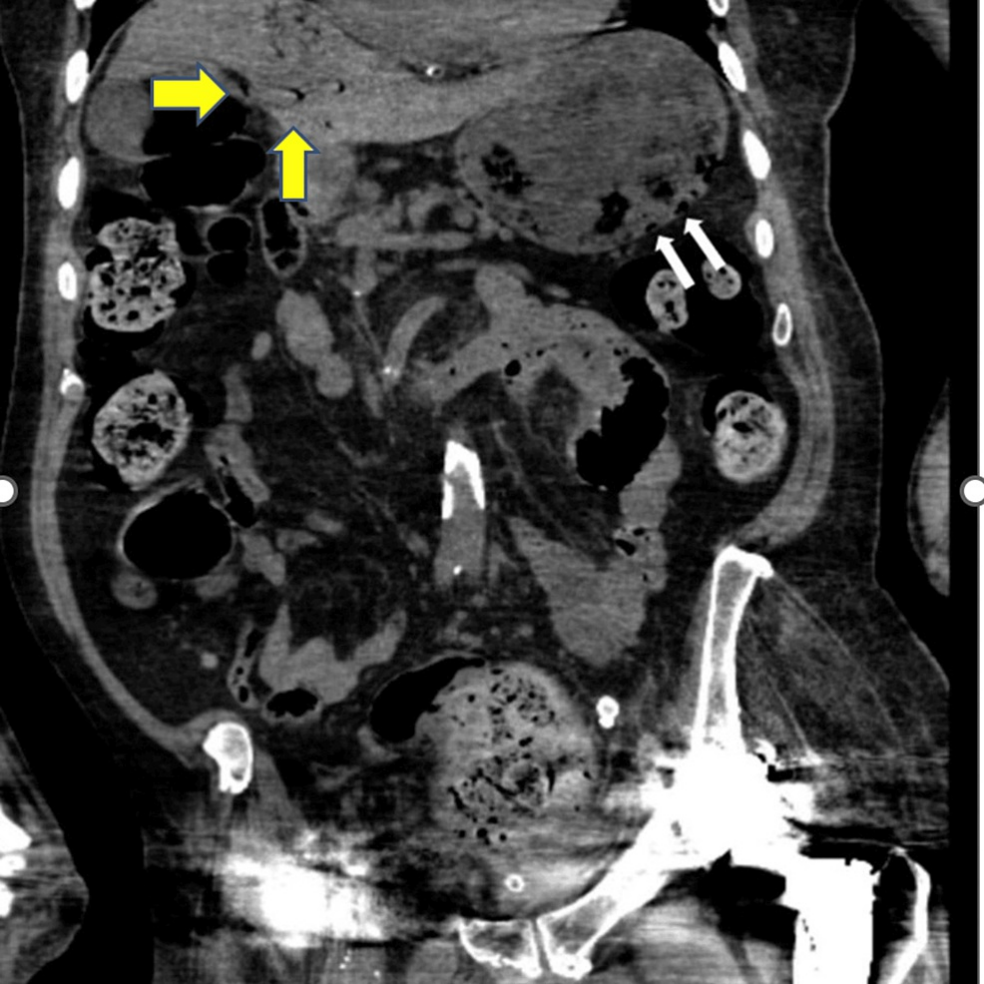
Coronal view of CT abdomen and pelvis without contrast White arrows: Distended stomach with questionable pneumatosis within the wall of the fundus; Yellow arrows: Portal venous gas within the liver
